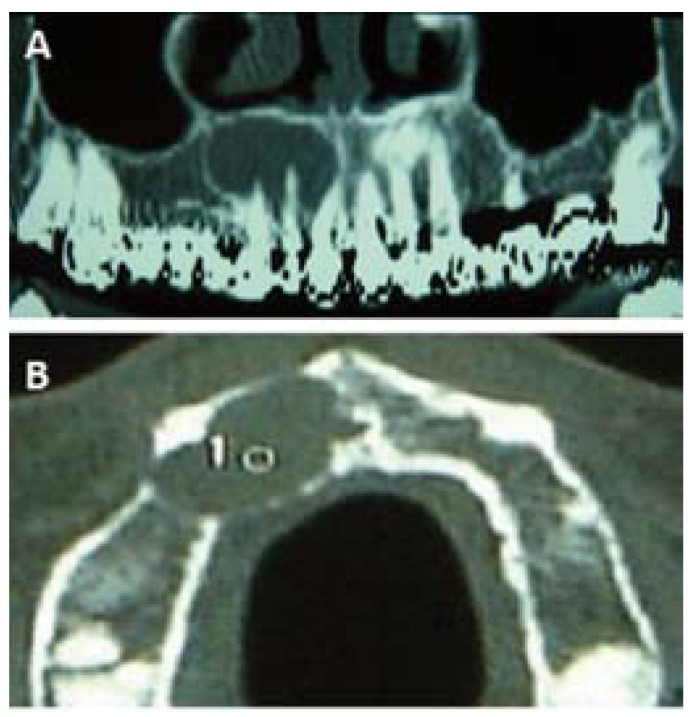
A) Tomographic reconstruction of the upper maxilla: Radiotransparency on the right side, displacing the floor of the nasal passages. B) CT. Axial view: Bulging of the vestibular and palatine cortical layers.
